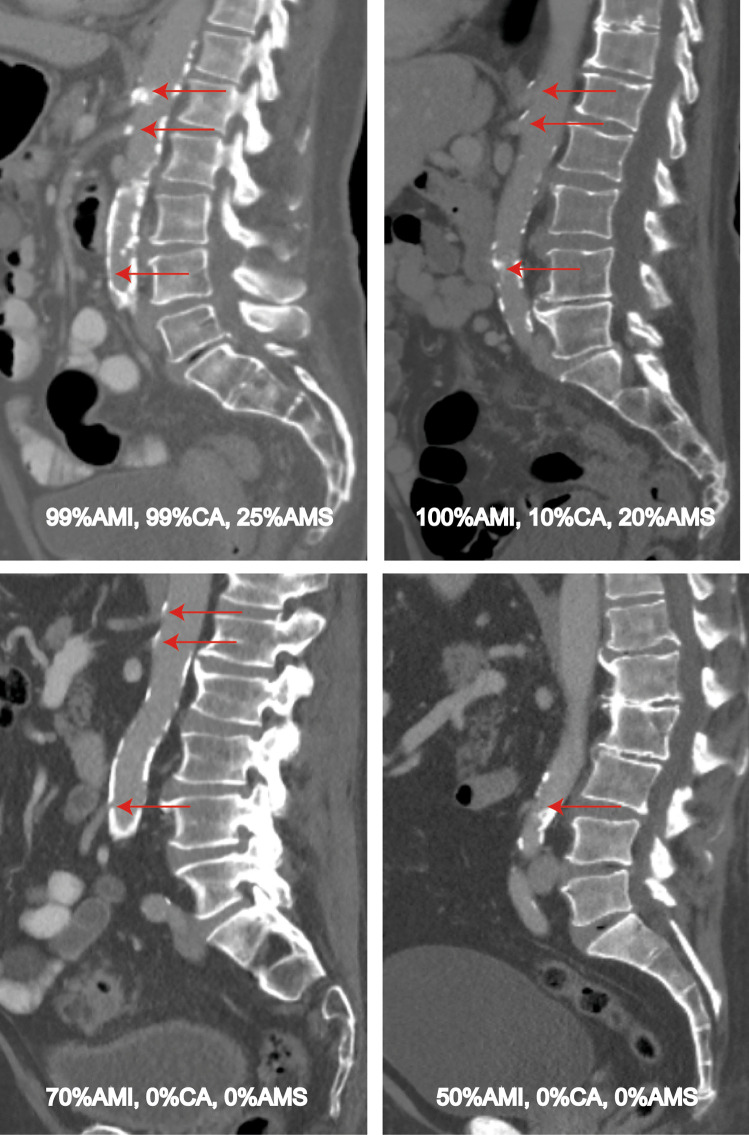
Abdominal CT-images of patients with mesenteric occlusive disease
